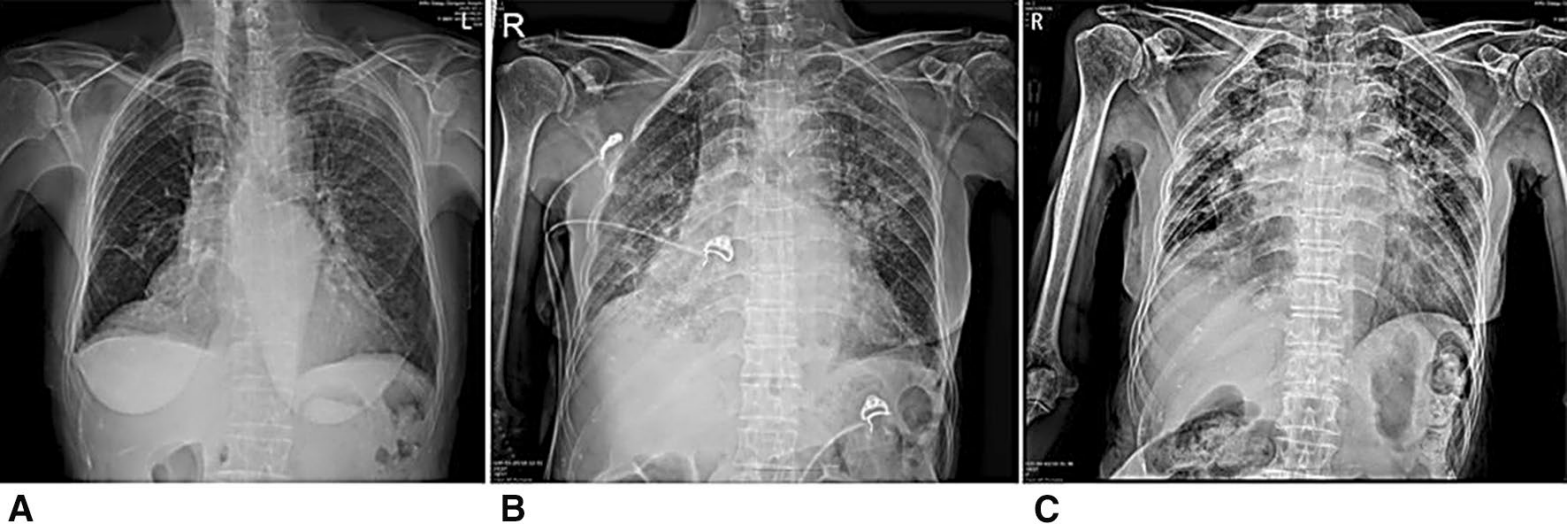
Serial chest radiographies of patient D. A Shows mass like consolidation at LUL, diffuse GGO at left lung, suggestive of COVID pneumonia at initial chest radiograph. B Taken 3 days after shows aggravation of pneumonia, or increased extent of pneumonia, diffuse GGO and consolidation at both lung fields. C Taken 10 days after shows slightly more aggravated pneumonia at both lung field
